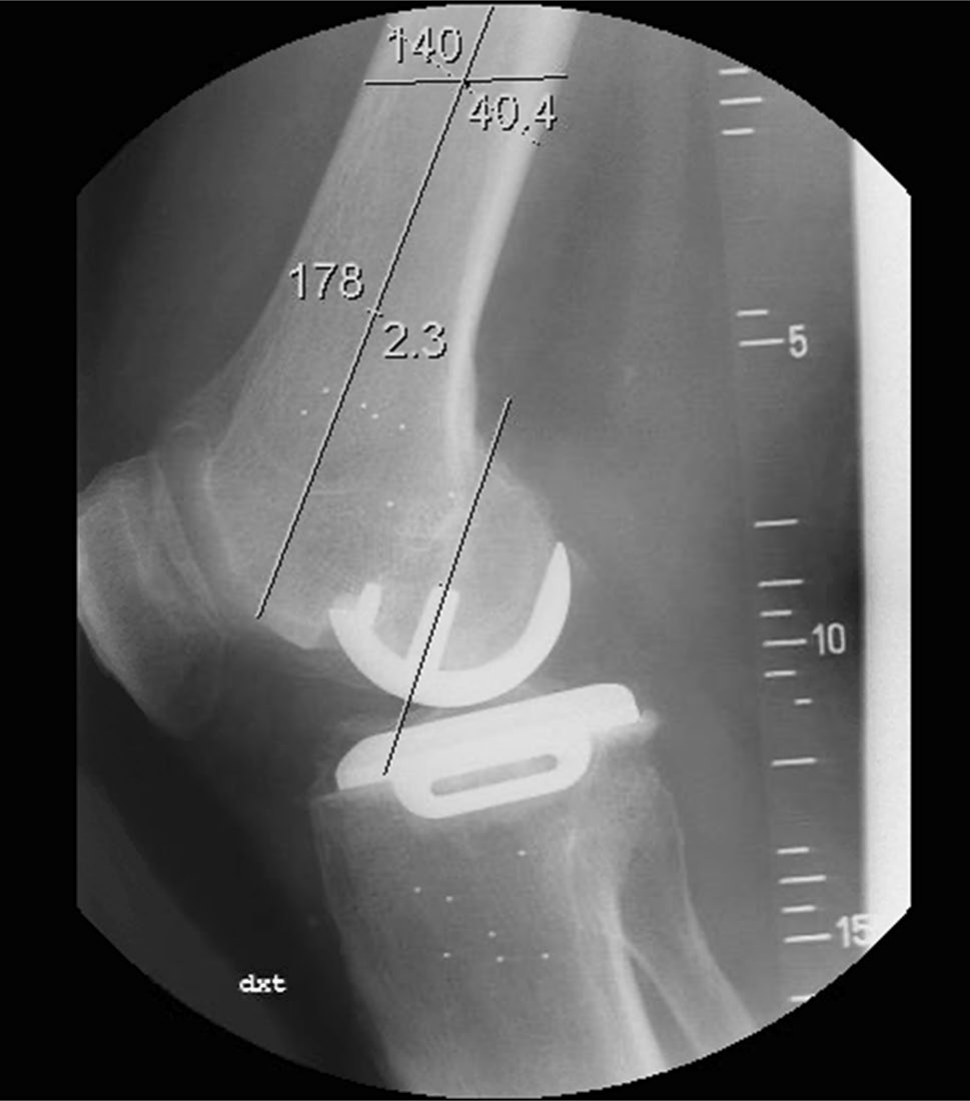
Flexion angle of the femoral component relative to the femoral bone axis measured on X-rays (positive = flexion, negative = extension)
